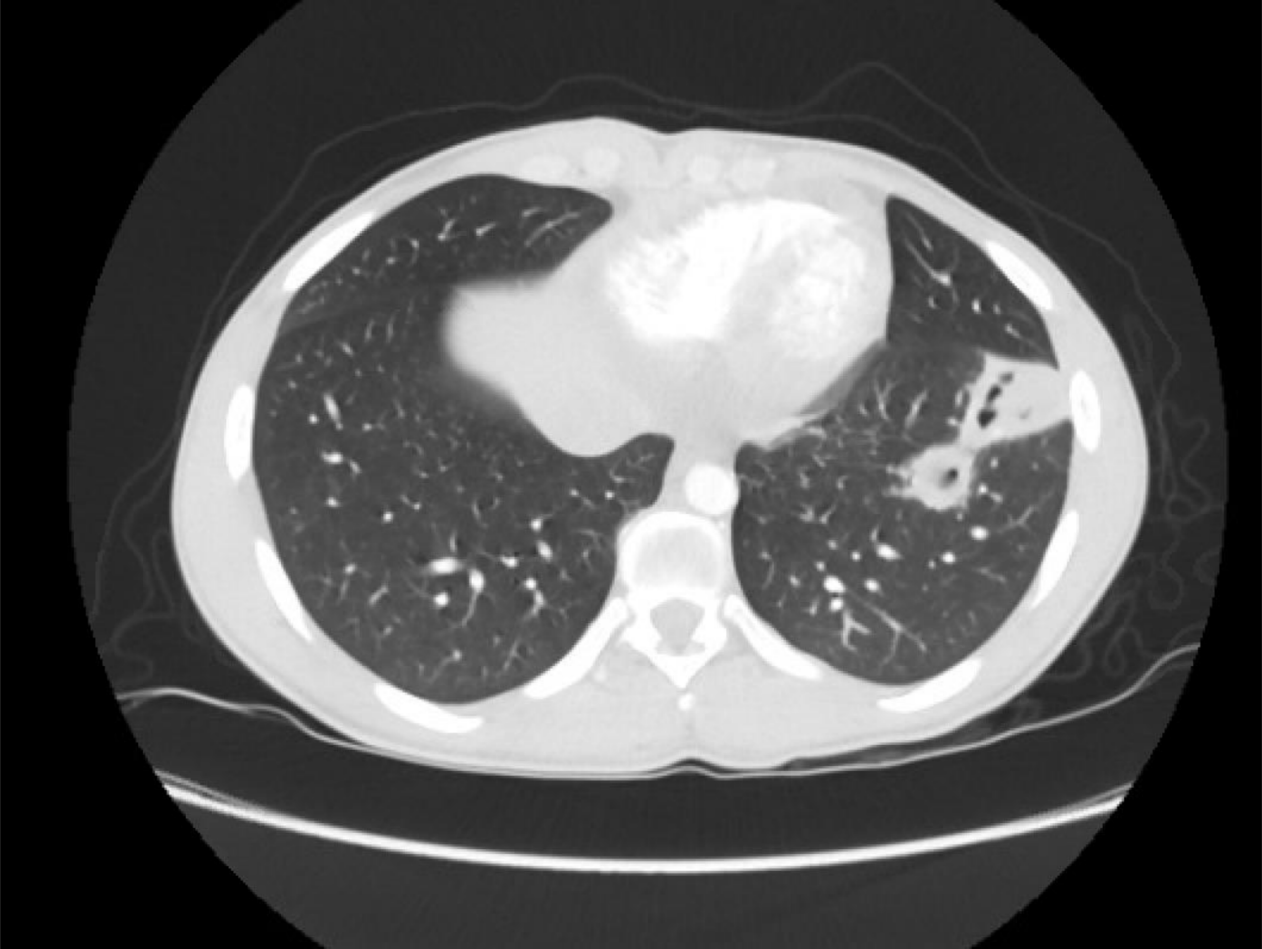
CT chest from Day 1 of 2nd admission showing airspace opacities with cavitation.
